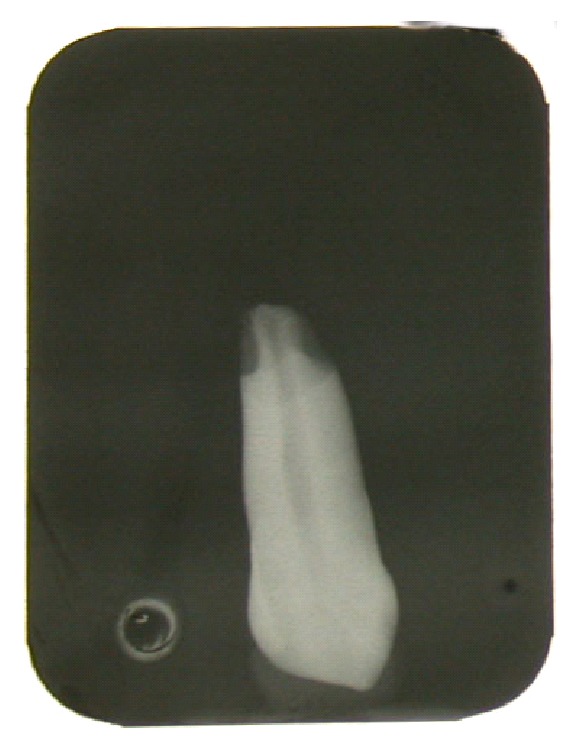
Traditional endooral X-ray: rizalysis.
